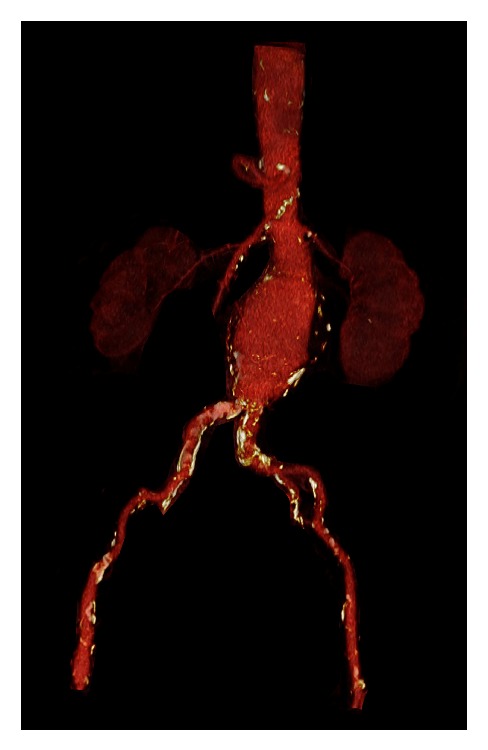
Preoperative angio-CT scan showing a 6 cm abdominal aortic aneurysm suitable for EVAR.
